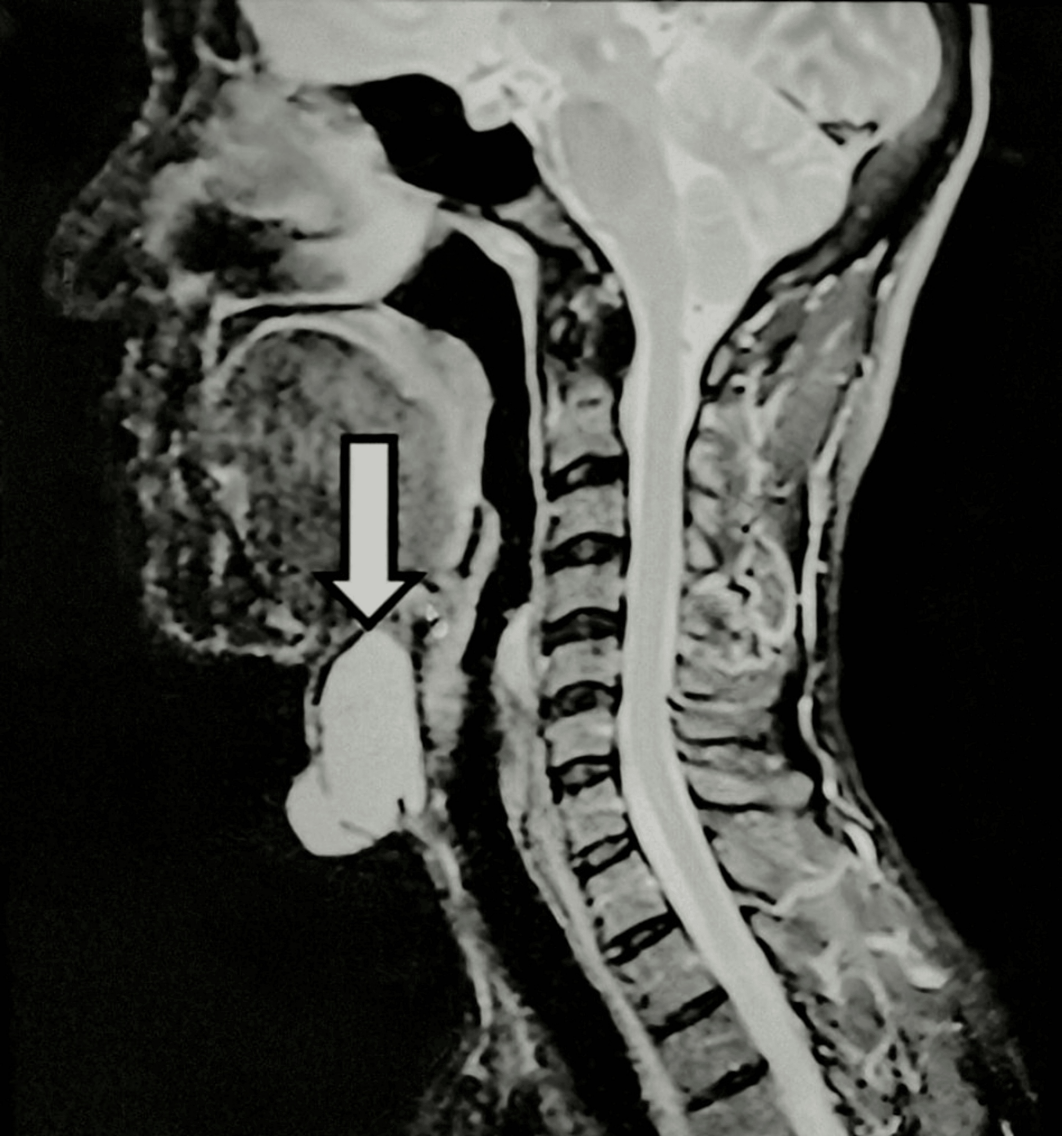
MRI neck (sagittal view) showing a well-defined cystic lesion in the midline of the neck (white arrow) that is hyperintense on T1- and T2-weighted images. There is no evidence of communication with the thyroid gland
